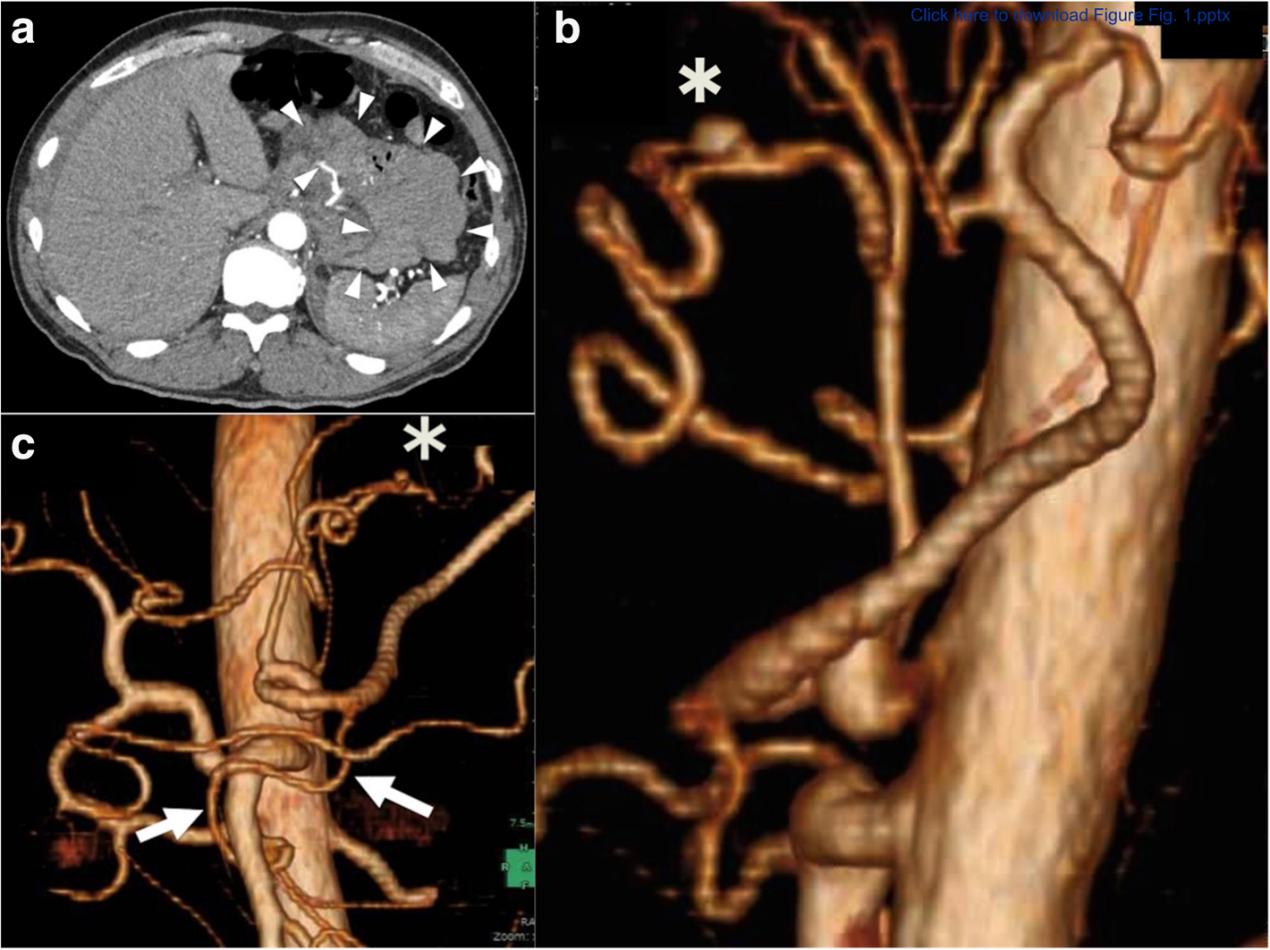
a. Enhanced contrast computed tomography (CT) shows a massive hematoma in the lesser omentum. b. In the lateral view of the aorta, the celiac axis is stenosed, most likely due to the median arcuate ligament (MAL). There is a right gastric artery aneurysm (*). c. 3D volume rendered CT shows a right gastric artery aneurysm (*) and an anomaly of the artery; the common hepatic artery branches from the superior mesenteric artery (SMA); the dorsal pancreatic artery (DPA) connects the SMA and the splenic artery (arrows)
